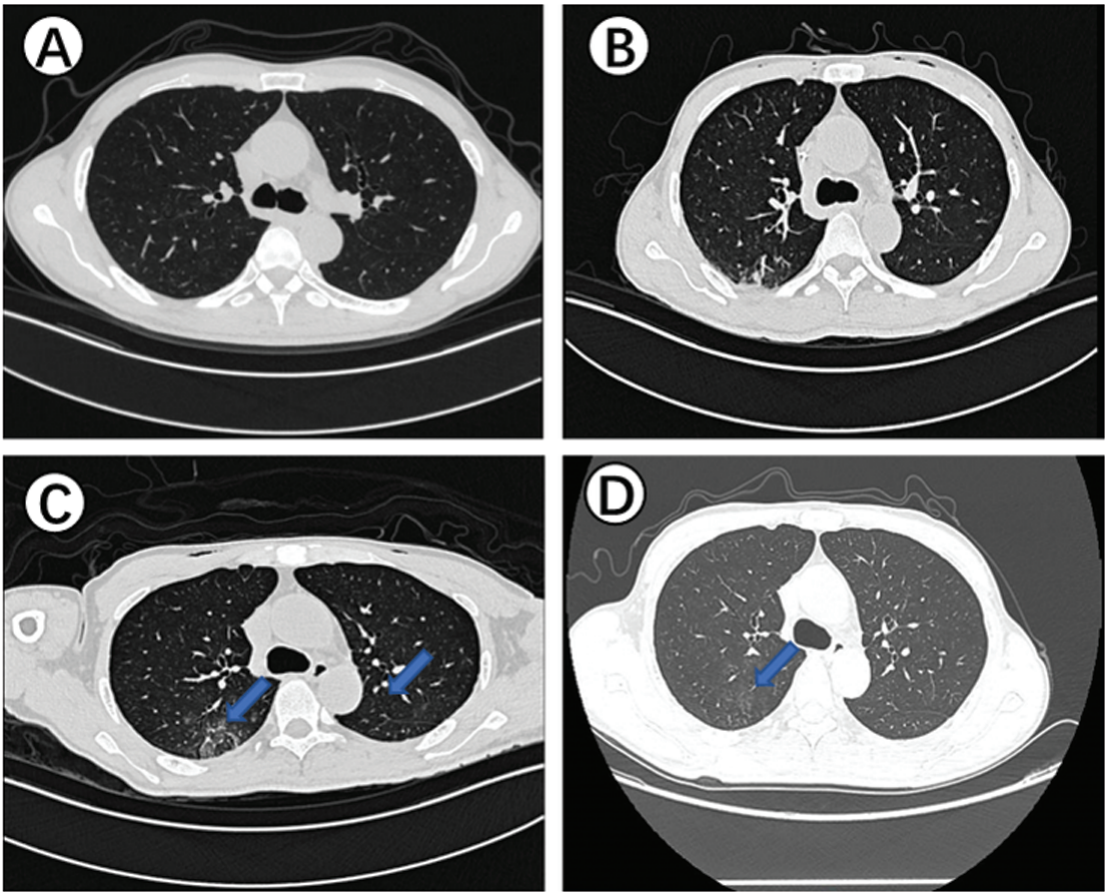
Chest CT of the patient. *A*, On day 2 before the operation, no abnormalities in the lung. *B*, On day 3 after the operation, infection was found in the bilateral lower lung fields but without radiologic characteristics of the SARS-CoV-2 infection. *C*, On day 7 after the operation, the blue arrows show the ground-glass opacities affecting bilateral, subpleural lung parenchyma, which are radiologic characteristics of the SARS-CoV-2 infection. *D*, On day 14 after the operation, the blue arrow shows the ground-glass opacities and the consolidation dissipated into irregular linear fibrosis and irregular fibrosis foci. Abbreviation: SARS-CoV-2, severe acute respiratory syndrome coronavirus 2.
